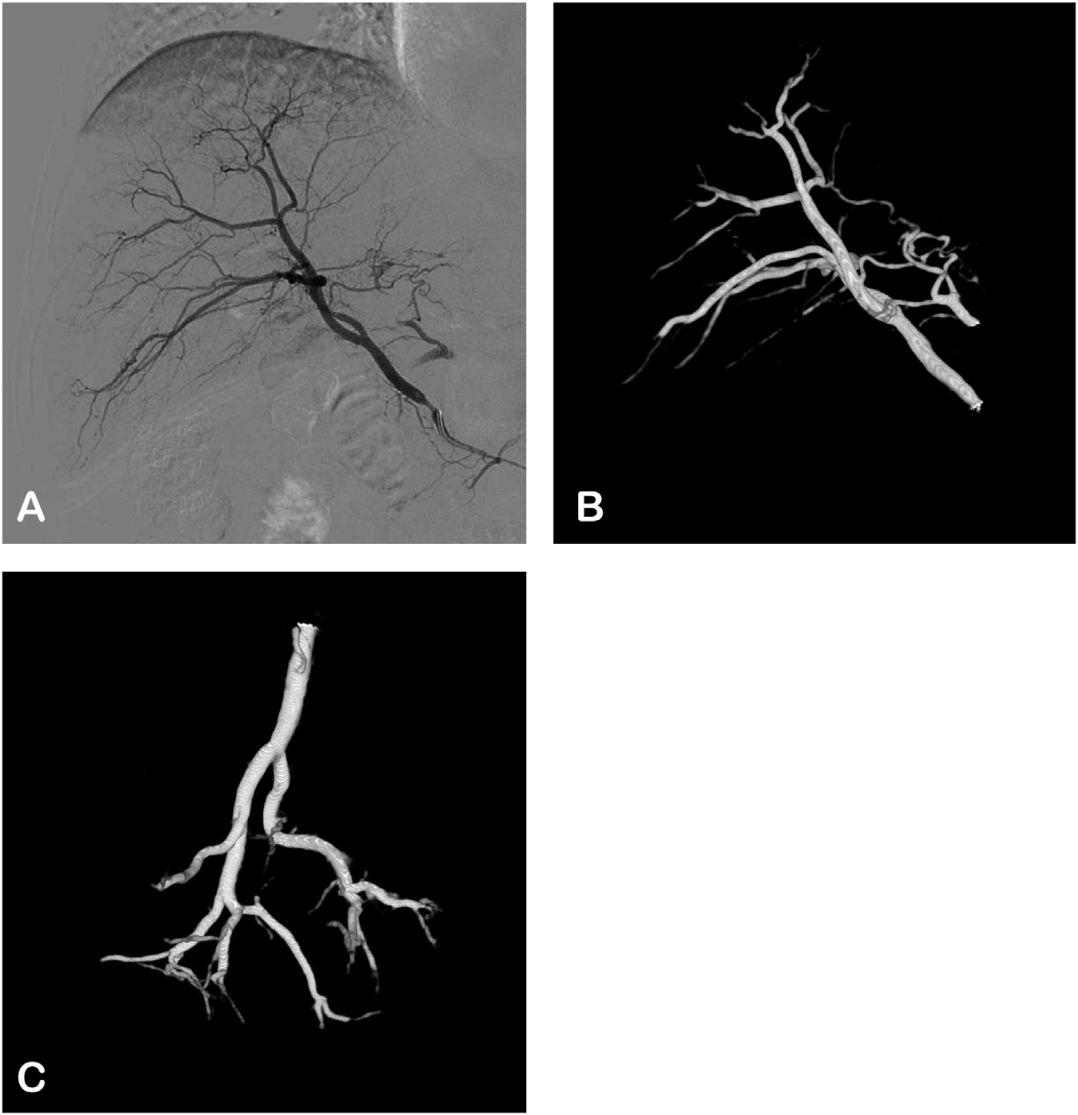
The angiographic images of the right hepatic artery in a 62-years-old male. (A) Digital subtraction angiography (DSA): postero-anterior view. (B,C) 3D views based on CBCT angiography.
